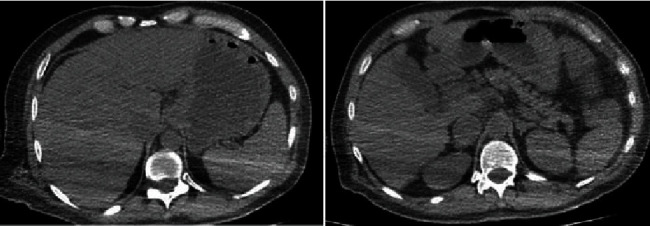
Abdomen CT scan showing a normal liver.
